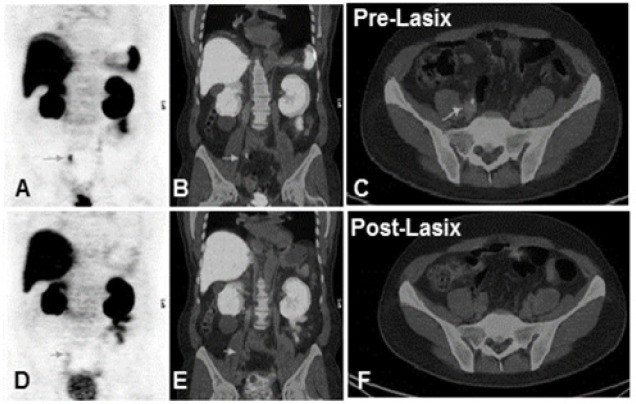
MIP and Fused PET/CT Images (A-C) Respectively, Showing Focal Uptake in the Ureter in Pre Lasix Study (Arrows) which Mimicked PMSA Avid Lesion. However, it was cleared in post Lasix images (D-F)
